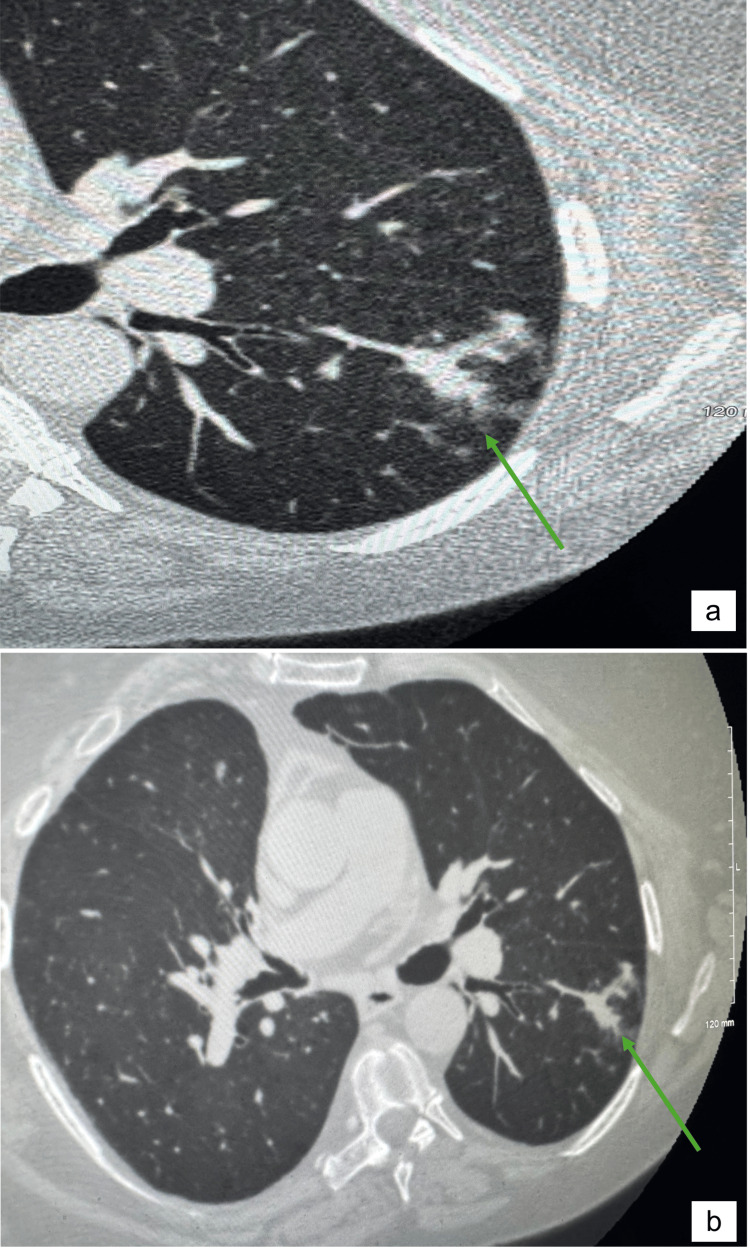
(a, b) CT chest with coned-down view taken on 12/16/19 shows irregular focal opacity in the superior segment of the left lower lobe of the lung (green arrows) CT: computed tomography
